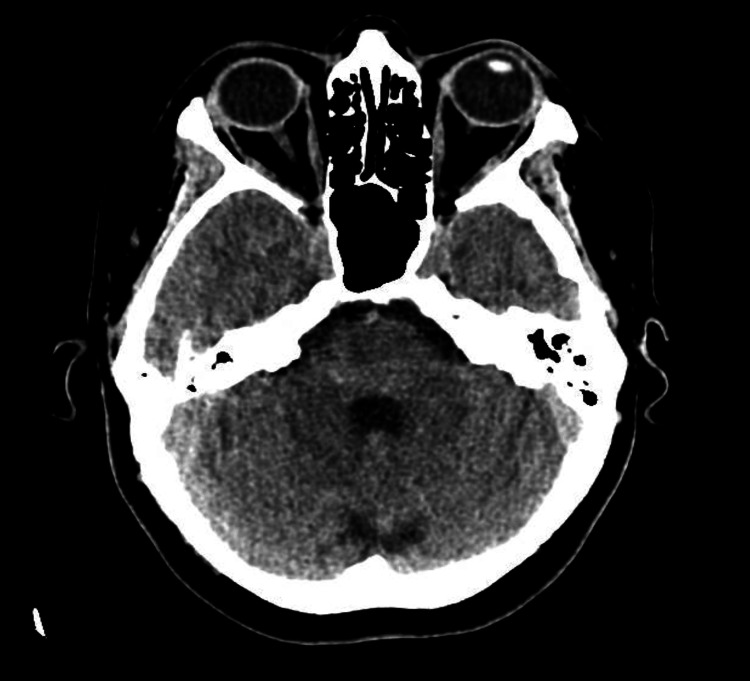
CT of the head. The ventricles and basal systems were patent, allowing for age-related cortical evolution with no disproportionate low atrophy. No acute intra- or extra-axial hemorrhage, large vessel territorial infarct, or parenchymal mass lesion was identified within the limitations of this non-contrast enhanced examination. No acute skull or base fracture was noted, with benign hyperostosis frontalis interna.
